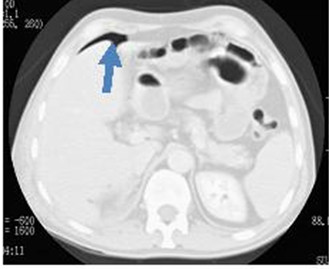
Abdominal CT scan with lung window shows free air adjacent to liver edge due to colon perforation.
